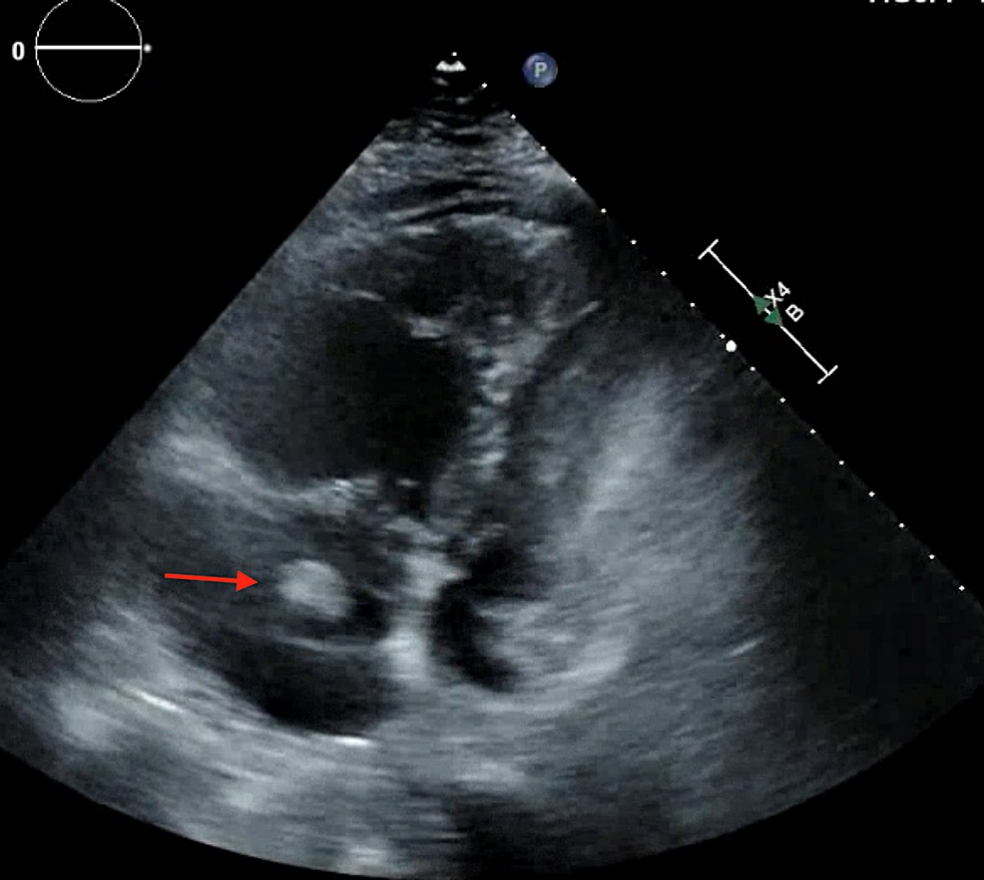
Transthoracic echocardiogram showing a thrombus in the right atrium (red arrow) and dilated right ventricle (apical four-chamber view).
